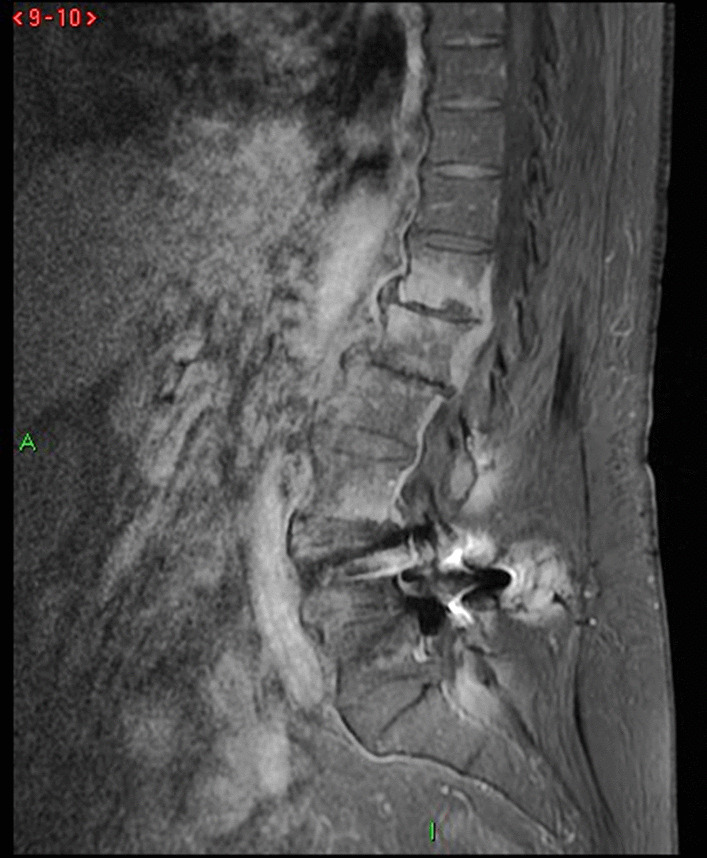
Sagittal injected magnetic resonance imaging showing high epidural and prevertebral soft tissue enhancement
